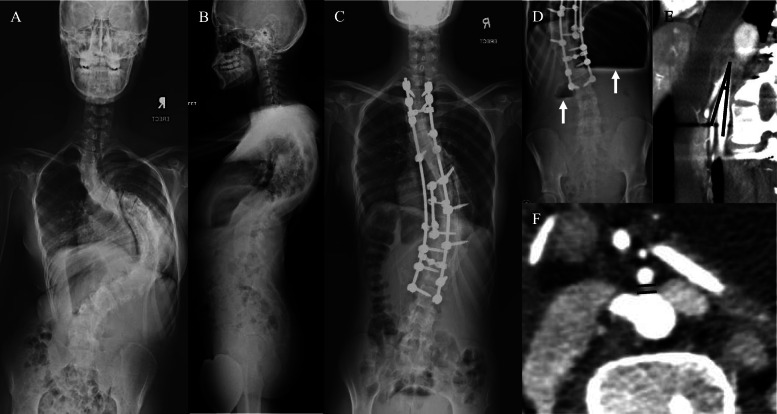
Another case with superior mesenteric artery (SMA) syndrome after scoliosis surgery (preoperative x-ray – a & b, postoperative AP x-ray – c) Erect abdominal radiograph (d) showed double bubble sign (white arrows). Sagittal (e) and axial (f) of CTA of the abdomen revealed an Aortomesenteric angle of 10° and SMA-aorta distance of 2.6 mm. Clinical assessment, MRI whole spine and CT thoracolumbosacral did not show any secondary causes for scoliosis
